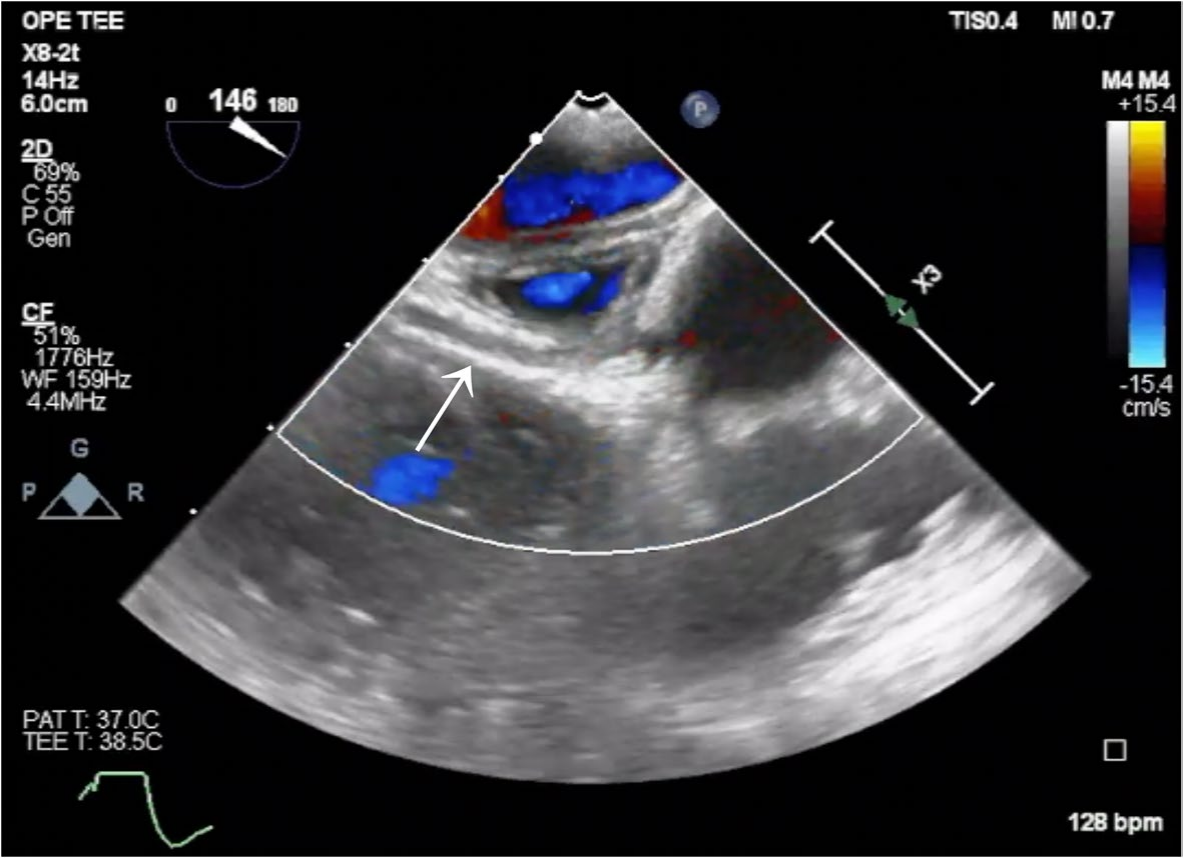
Absence of flow in the left circumflex artery Color Doppler image obtained using transesophageal echocardiography. The solid arrow indicates the left circumflex artery (LCx), where no color flow is observed
